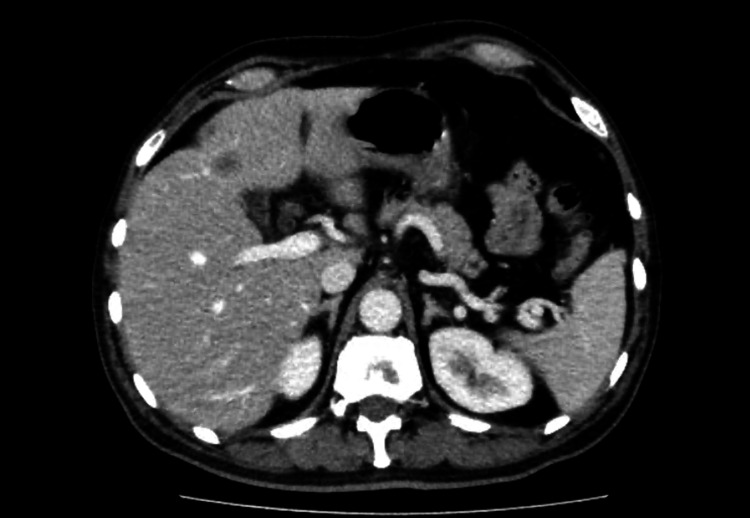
Abdominal CT scan showing new hepatic metastases (Case 2) De novo hepatic lesions.
